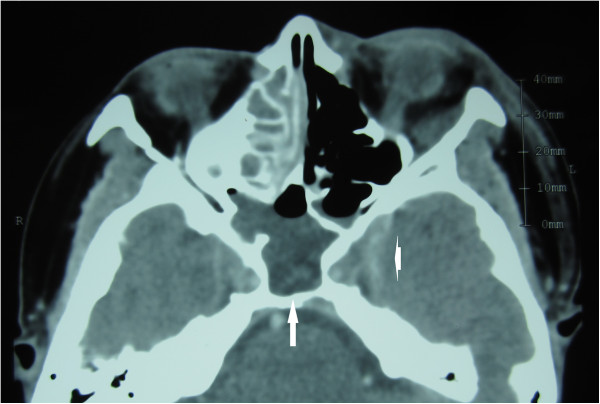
Contrast-enhanced CT. Contrast-enhanced CT showed enhancement and dilation of left cavernous sinus (arrowhead). Sphenoid sinus occupied the low density area. The right sphenoid sinus extended posteriorly to the left sinus and was in contact with both cavernous sinuses (arrow).
